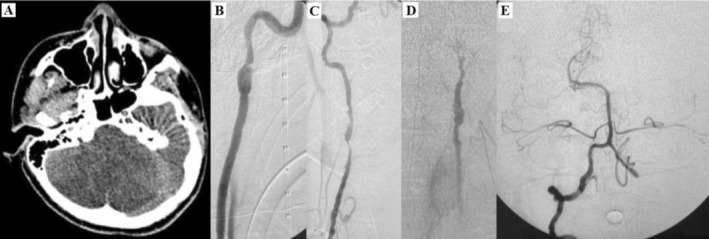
Head CT demonstrates diffuse hypoattenuation of cerebellar hemispheres (A). CTA identified luminal irregularities, prompting further investigation. Angiography reveals dissections in the right internal carotid artery at the craniocervical transition (B), V2 and V3 segments of the right vertebral artery (C), dysplastic V2 segment of the left vertebral artery with distal occlusion (D), and intracranial occlusions in the right posterior inferior cerebellar artery and V4 segment of the left vertebral artery (E). These findings are consistent with multifocal fibromuscular dysplasia.
